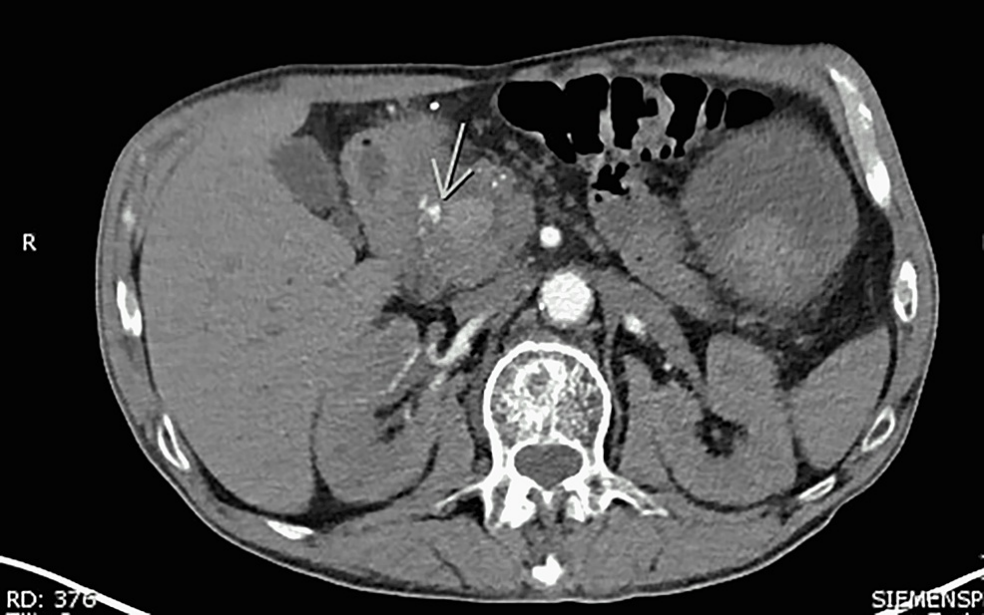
CT angiography of the abdomen and pelvis Axial CTA image of the abdomen and pelvis showing active extravasation into pancreatic pseudocyst (green arrow).
